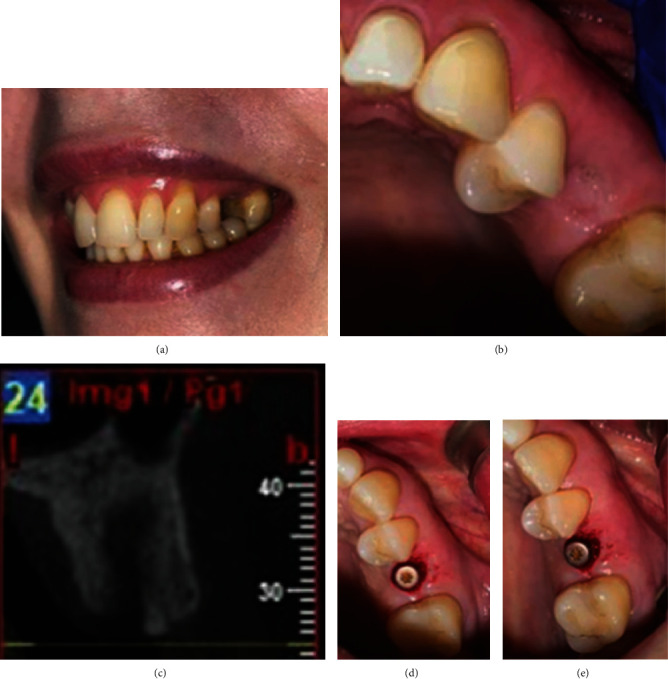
Lateral view upon smiling (a), occlusal view of extraction site of tooth 25 after 8 weeks (b), B–P radiographic section with root fragment in situ (c), and occlusal and buccal views of the healing abutment and remaining root shield immediately following implant placement (d and e).
